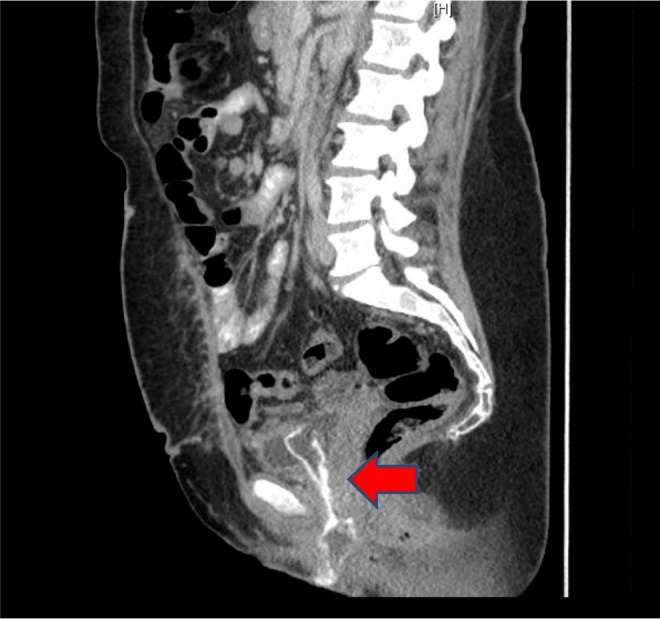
CT abdomen and pelvis sagittal section. High attenuation lesion in the urethra and bladder lumen (*arrow*).
